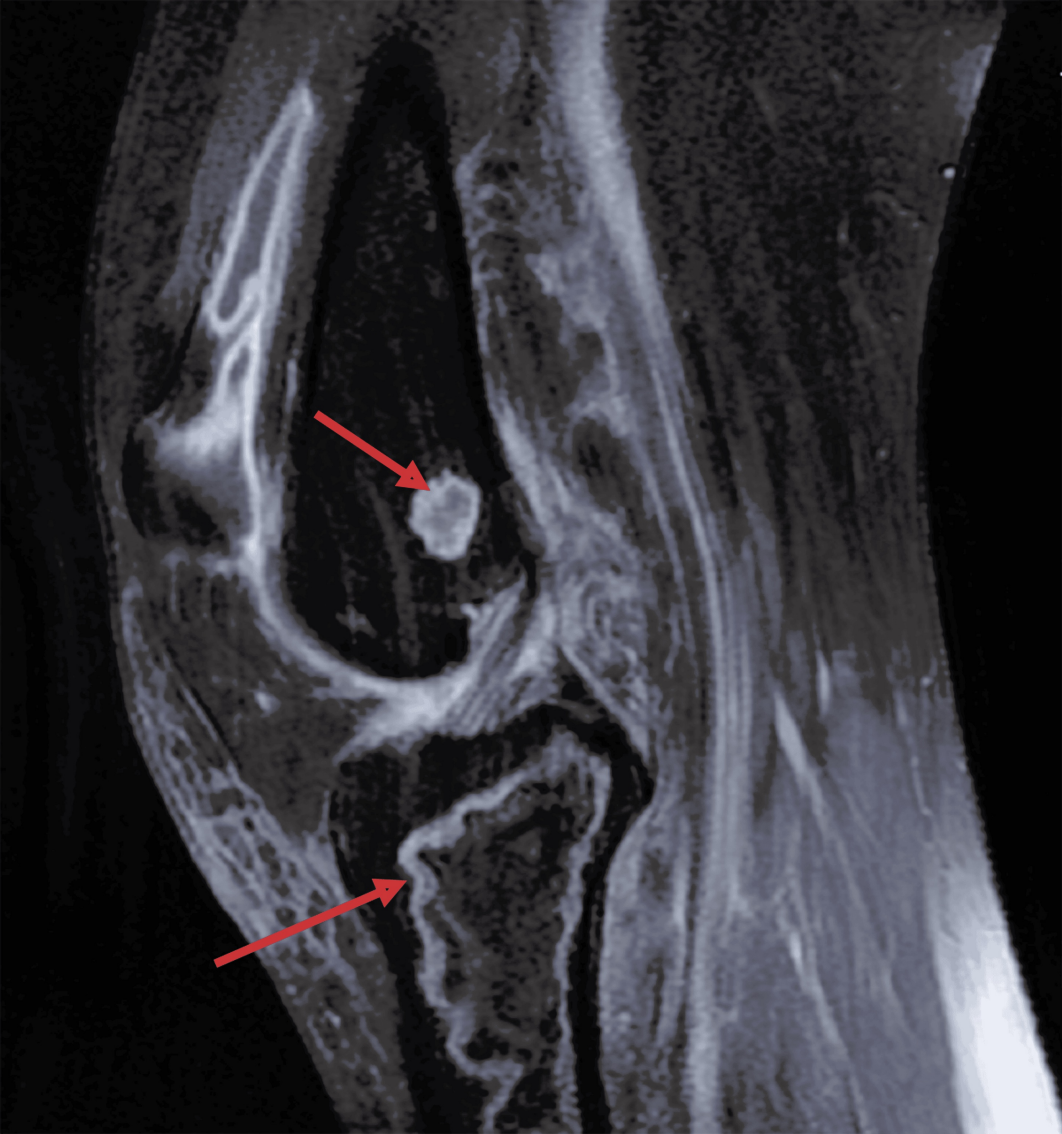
T2 fat-saturated sagittal MRI of the left knee showing osteonecrosis of both femur and tibia. Medullary meta-epiphyseal lesions in the proximal tibia and distal femur, exhibiting a serpiginous border and a double-line sign.
